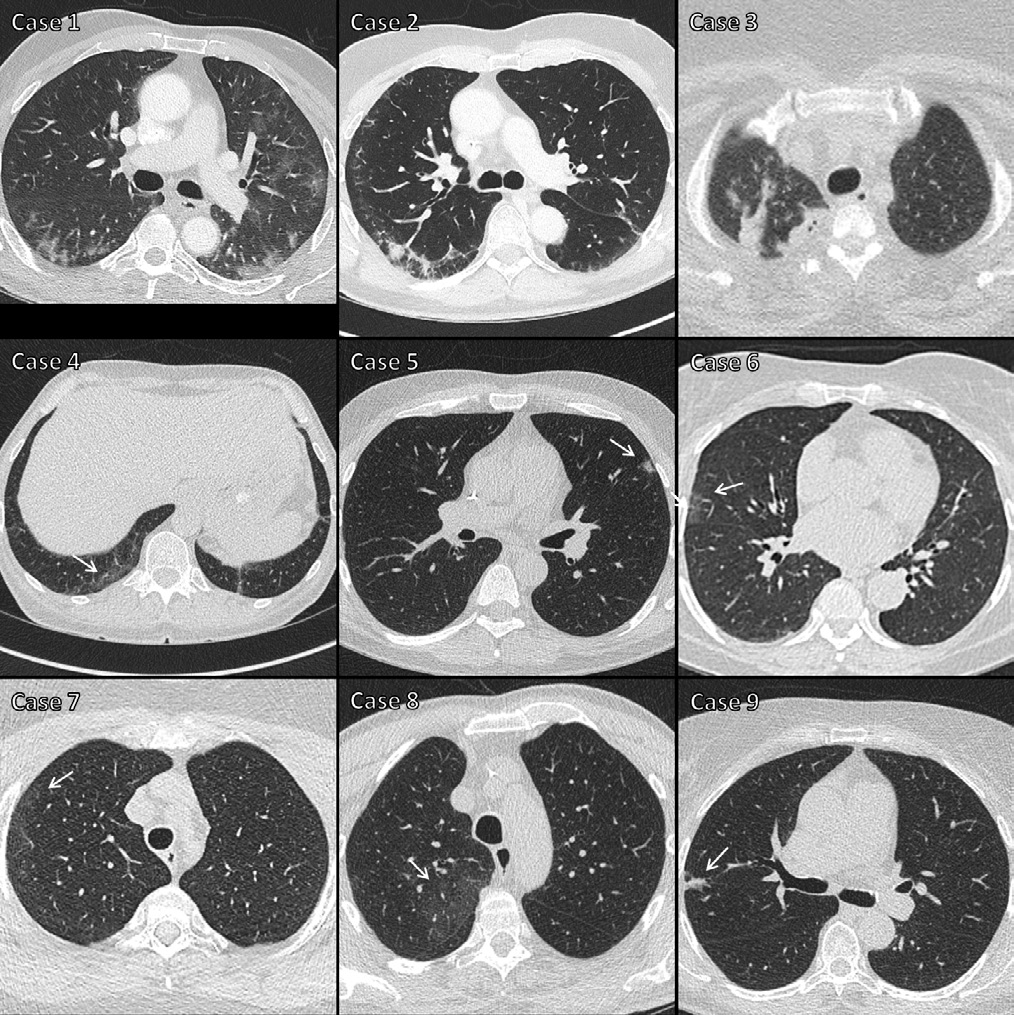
Images from the nine cases with “positive” CTs. The nine cases with “positive” CT results correspond to those listed in Table 4. Cases 1–3 were regarded as true-positive CT results, with areas of peripheral consolidation and/ or ground glass density, indicating either “probable COVID-19” or “classic COVID-19”. Cases 4–9 were regarded as false-positive CT results, with either very limited extent of ground glass opacity, or features more suggestive of a non-COVID-19 process (arrows).
